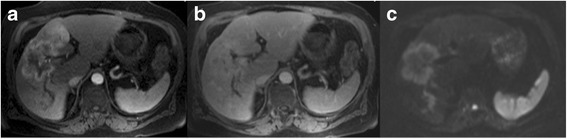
LR-M a Arterial phase MRI showing peripheral hyperenhancement. b Delayed phase image (at 5 min) showing central enhancement. c At DWI a targetoid appearance is apparent. Image features are not in line with HCC, but indicative of LR-M. Lesion was proven to be CCC
